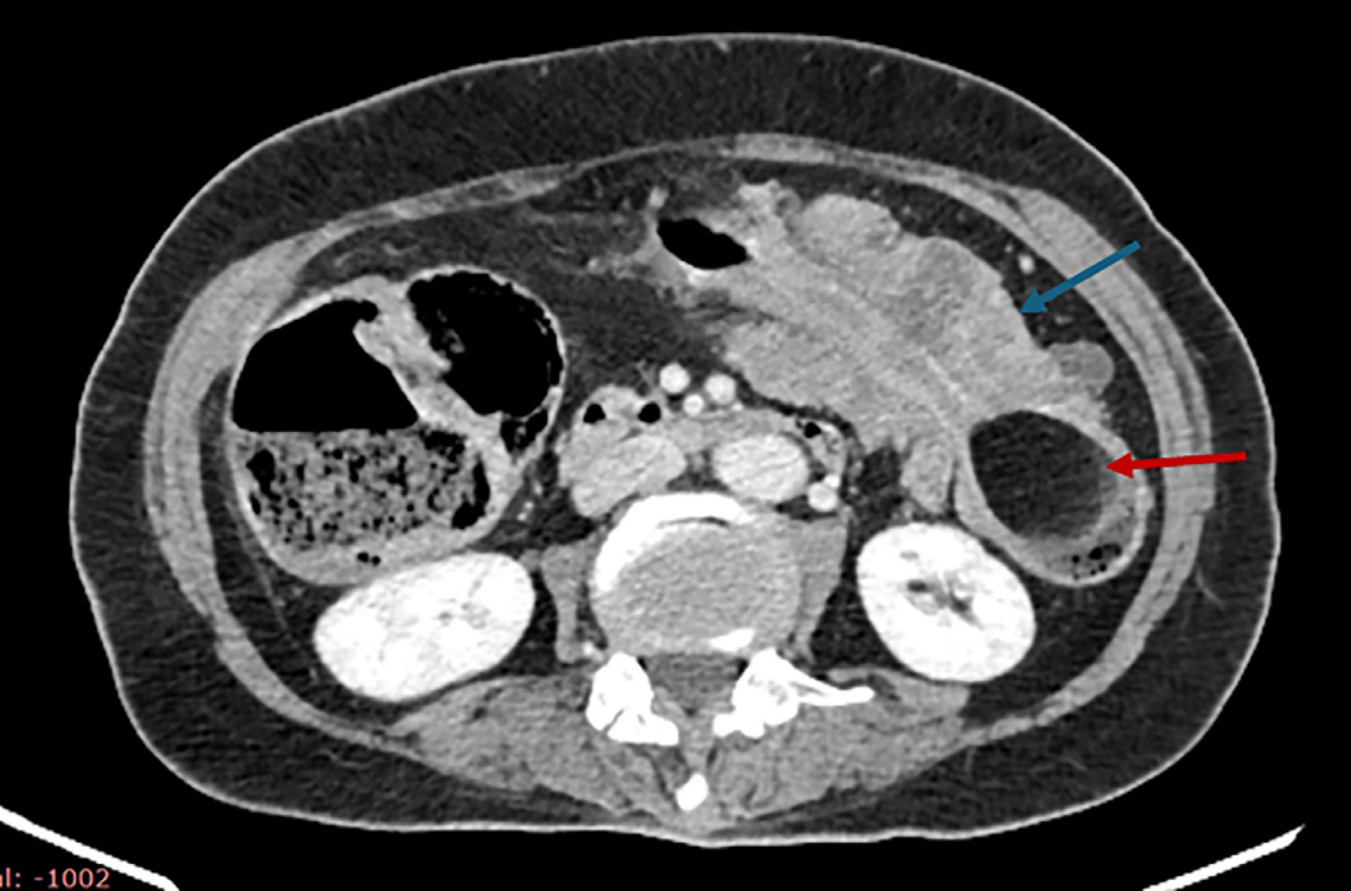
Axial contrast-enhanced CT showing colocolic intussusception (blue arrow). The previously identified fat-density lesion remains nonenhancing, further supporting its benign nature as a submucosal lipoma acting as the lead point (red arrow).
